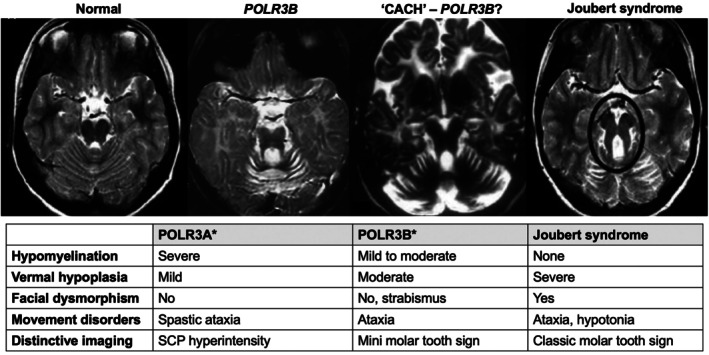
From “mini‐molar tooth” to classic “molar tooth” sign. Axial T2‐weighted brain MRIs showing, from left to right, normal midbrain; *POLR3B*‐associated ataxia (sister 1 at 19 years of age as originally reported), childhood ataxia with cerebral hypomyelination (CACH, undiagnosed case report of three young siblings most likely associated with a *POLR3B* mutation), and a the classic “molar tooth” sign (within black circle), typical of Joubert syndrome. Compared with the classic molar tooth sign of Joubert syndrome, the “mini molar tooth” sign exhibits thin rather than thick superior cerebellar peduncles and a relatively shallow rather than deep anterior foramen cecum. * Both POLR disorders yield variants of an “4H” imaging phenotype: Hypomyelination, hypodontia, and hypogonadotropic hypogonadism. SCP: Superior cerebellar peduncle. Permission was granted to republish second^1^, third^2^ and fourth MRI^3^.
